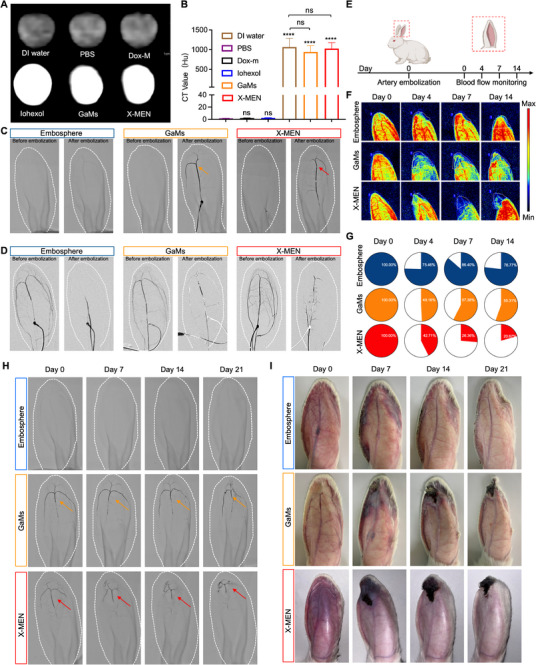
High‐resolution imaging and embolization effect of X‐MEN. (A) CT images of DI water, PBS, Dox‐m, Iohexol, GaMs, and X‐MEN. (B) CT values of DI water, PBS, Dox‐m, Iohexol, GaMs, and X‐MEN. Significant differences were evaluated by a two‐tailed unpaired *t‐*test. Data are presented as means ± SEM, *n* = 3, ns *p* > 0.05, ^^
